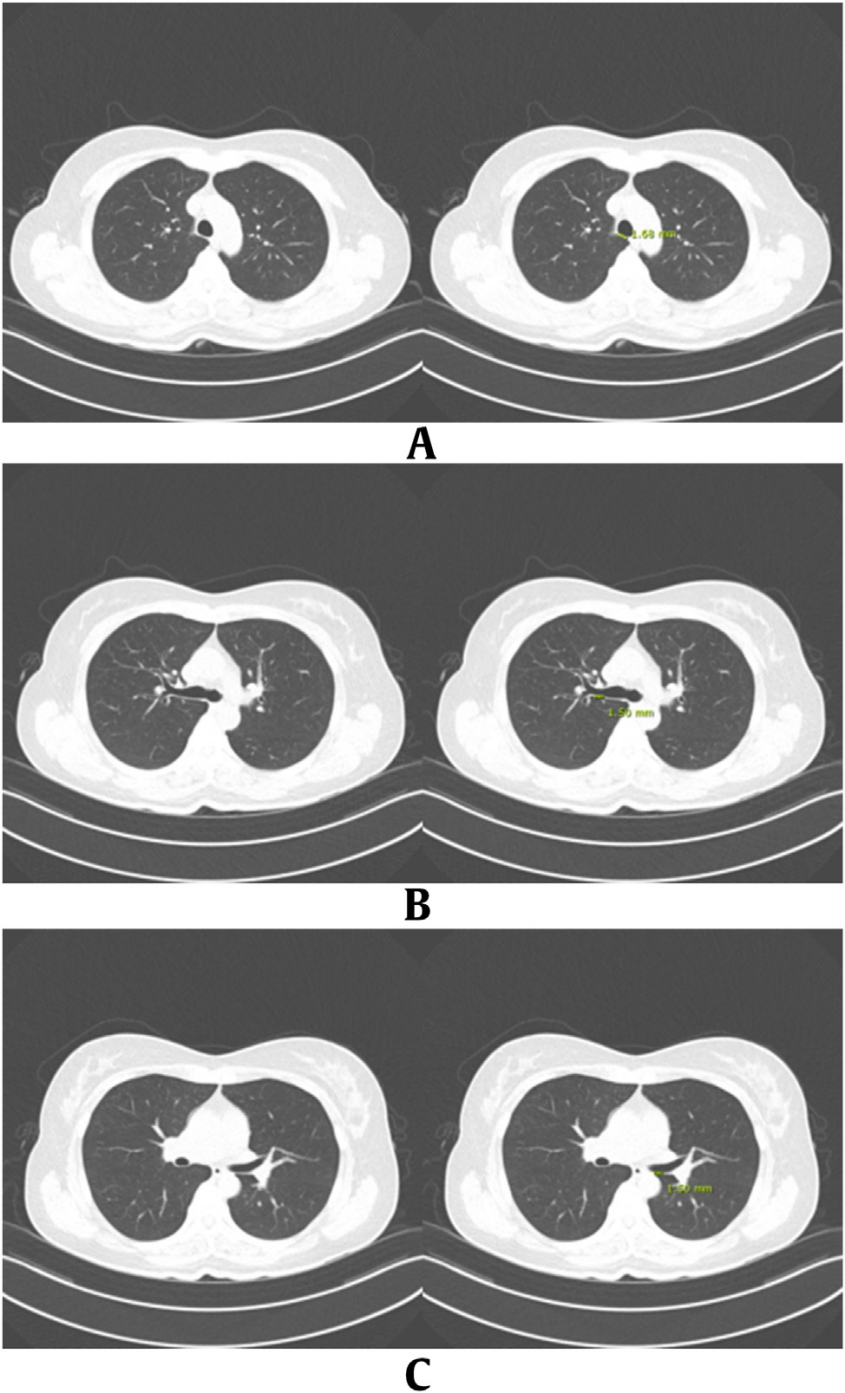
Methods of measuring bronchial wall thickness on CT image. Bronchial wall thickening was measured at three levels with most distinct location with minimal motion artifacts: (A) the trachea, just above to the azygos vein, (B) the right upper lobar bronchus, about 2–3 cm distal from the tracheal bifurcation, and (C) the left main bronchus, at the area positioned between the esophagus and the left lower lobar pulmonary artery, ensuring that no adjacent soft tissue structures interfered with the assessment of wall thickening. CT, computed tomography.
